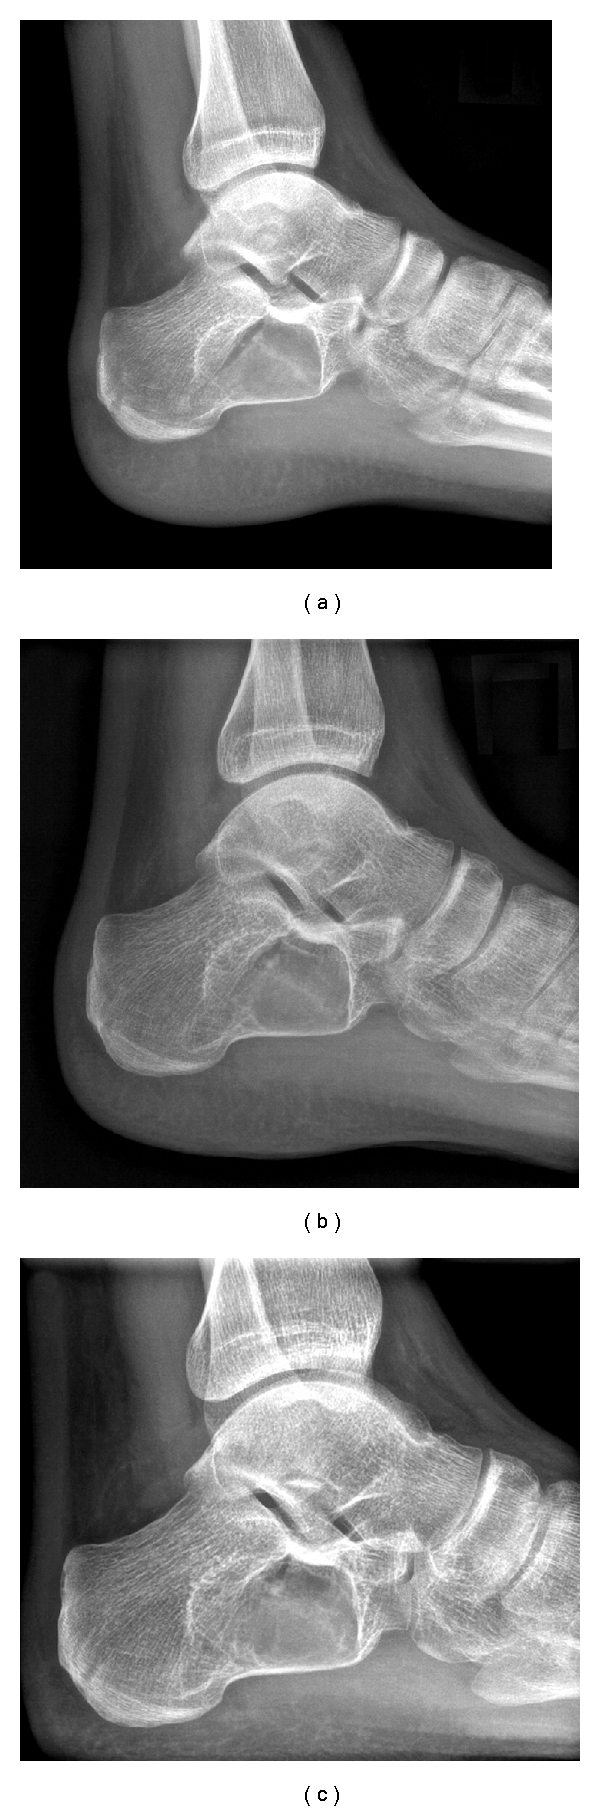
(a)Radiographic control 4 weeks after injury. (b)Radiographic control 8 weeks after injury. (c)Radiographic control 18 months after injury.
